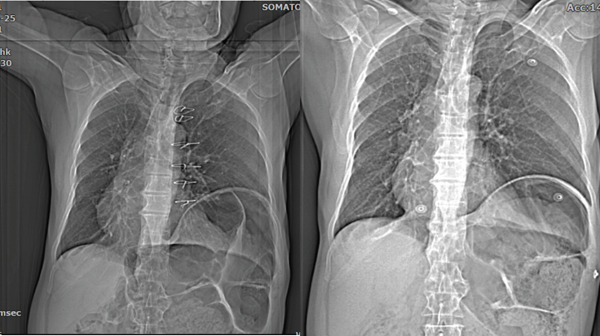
Chest CT scan (coronal view). Dynamic heart position with post‐prandial, 2 years post operation (left) and pre‐prandial, pre operation (right).
